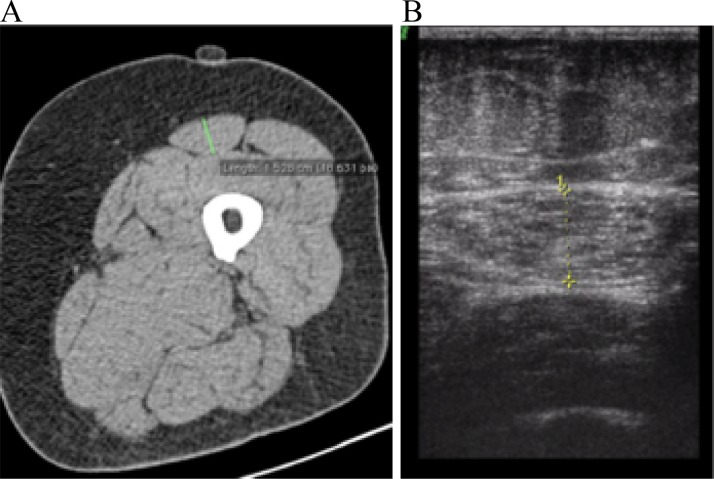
RF_CSA_ evaluation by CT (A) and US (B). RF_CSA_: Cross-sectional area of the rectus femoris; CT: Computed tomography; US: Ultrasound. Continuous green line: Rectus Femoris diameter as measured by CT; dotted yellow line: RF_CSA_ as evaluated by US
